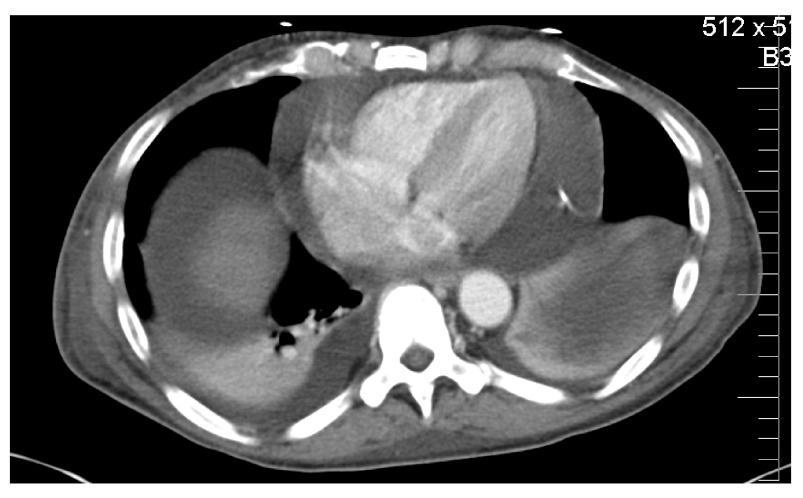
CT Chest. Large pericardial and pleural effusions. The tip of the drain is shown in the pericardial space.
